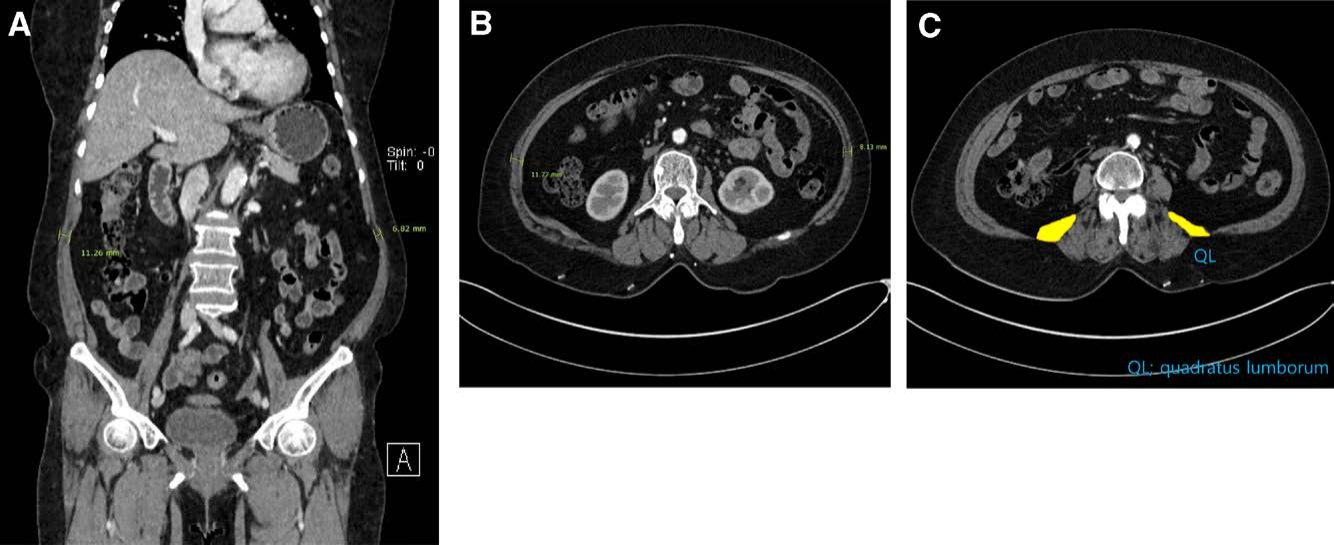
Computed tomography findings of the abdomen and pelvic cavity showing a reduced thickness of the left abdominal wall muscle and quadratus lumborum. (A) Coronal image. (B) Axial image. (C) Reduced cross-sectional area of the left quadratus lumborum.
